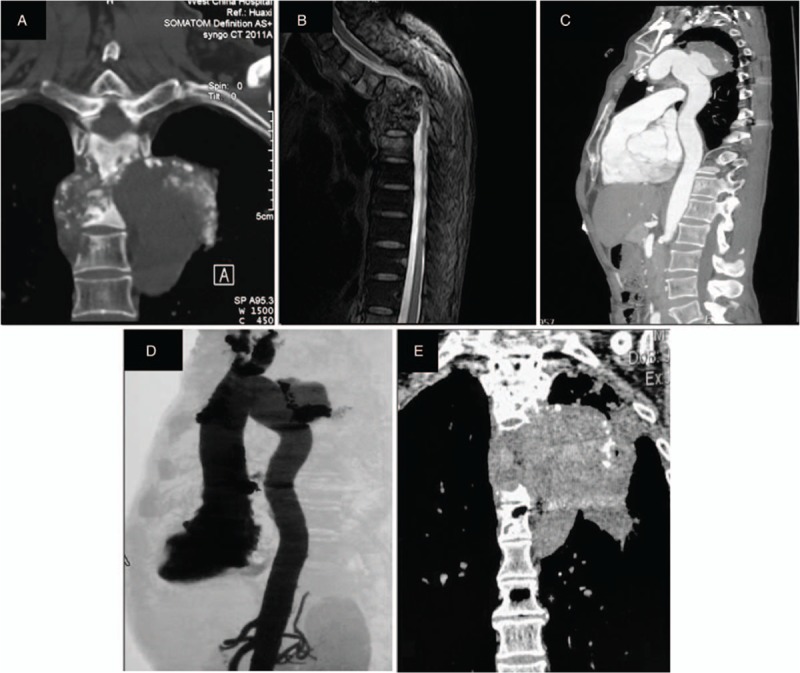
Patient 1: CT (A) and MRI (B) reveal multiple bone destruction of T2–T6 vertebrae with peripheral abscess formation. Enhanced CT (C) and angiography (D) show the descending aorta aneurysm around the T4 and T5 level. CT shows the paravertebral mass has expanded in the end of follow-up (E). CT = computed tomography, MRI = magnetic resonance imaging.
